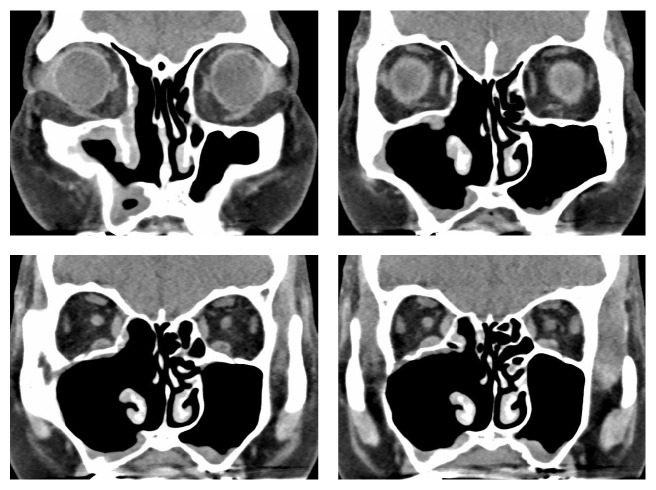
Postoperative CT observation. Granulation-like mucosal edema remained, but the maxillary sinus was widely opened from the middle and inferior meatus sides. The inferior turbinate was preserved.
